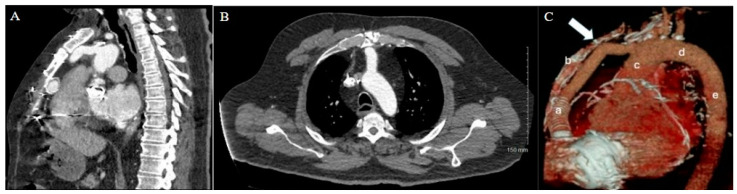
Patient 1 CT chest. (A) Sagittal view and (B) axial view reveal extensive adhesions between LVAD outflow graft and superior retrosternum. (C) 3D reconstruction with labeled structures LVAD outflow graft (a), sternum (b), ascending aorta (c), aortic arch (d), and descending aorta (e) highlighting the point of maximal LVAD graft–sternal contact (white arrow).
